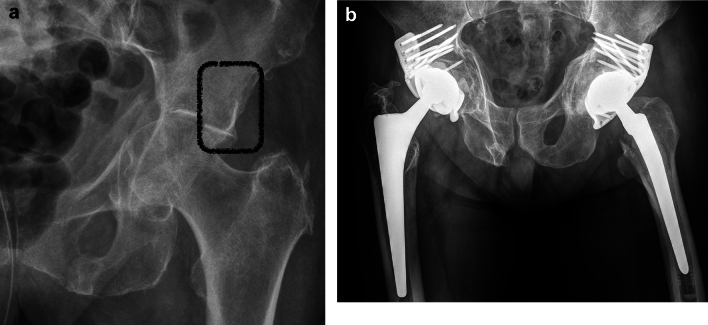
a ACPHT fracture with gull sign. The superolateral dome is intact and in osseous continuity with the supracetabular bone and the iliac wing. b Primary arthoplasty of ACPHT fractures using angular stable reinforcement rings. X-ray control after five years (right) and one year (left)
